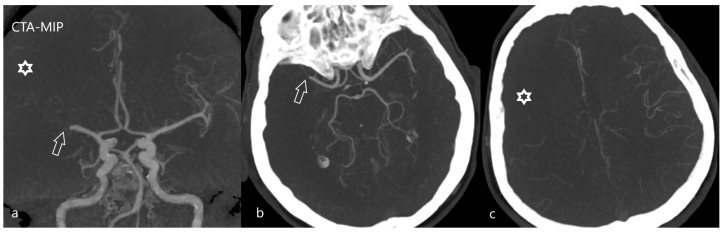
Malignant type of collaterals. CT angiography with maximum intensity projection reconstruction (CTA-MIP) depicts malignant collaterals in a patient with the occlusion of the right MCA [(a,b)—arrow]. Leptomeningeal collateral vessels are insufficient, peripheral arterial branches are not visible [(a,c)—star; compare with normal filling of vessels on the healthy left side].
